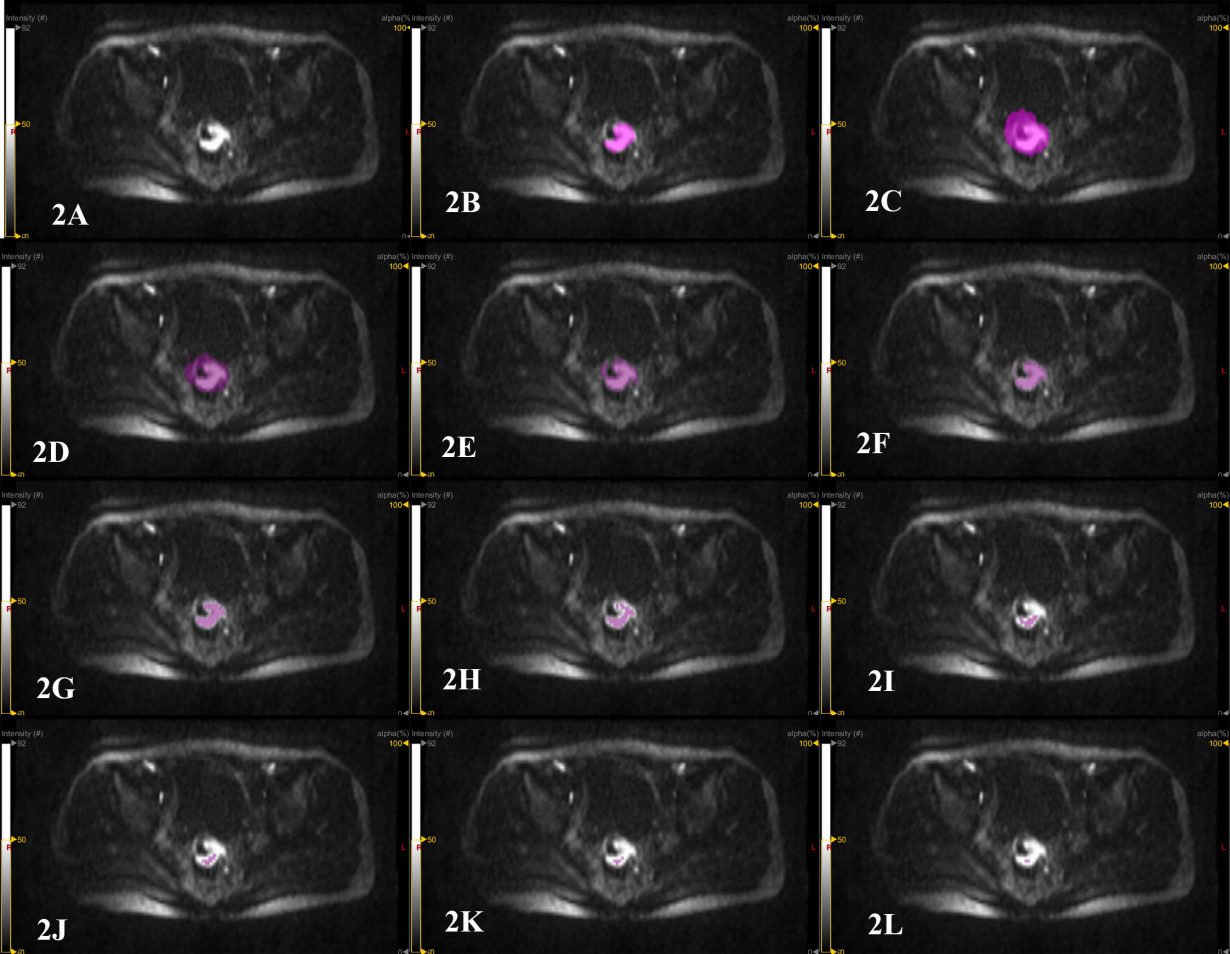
Patient, male, 67 years old, with distal rectal cancer. (A) the original image of the primary rectal cancer on a certain layer of the DWI image with b=1500 s/mm^2^. The high signal area is the tumor area. (B) manual delineation of the primary lesion of rectal cancer on this layer using the pencil 2D tool in the LIFEx software to measure GTV. The LIFEx software shows that the volume of the rectal tumor on this level is 4.078 cm^3^. After the lesion is delineated layer by layer, the LIFEx software automatically integrated the tumor volume of each layer, and the GTV was 22.25 cm^3^. (C) semi-automatic delineation of the primary lesion of rectal cancer by using the circle 3D tool of LIFEx software to cover the entire area of the primary rectal cancer. (D–L) semi-automatic delineation of tumor lesions using 10%, 20%, 30%, 40%, 50%, 60%, 70%, 80% and 90% of the highest signal intensity in the area as thresholds. The measured GTV were 70.29 cm^3^, 34.52 cm^3^, 19.16 cm^3^, 10.52 cm^3^, 5.564 cm^3^, 1.813 cm^3^, 0.705 cm^3^, 0.252 cm^3^ and 0.101 cm^3^.
